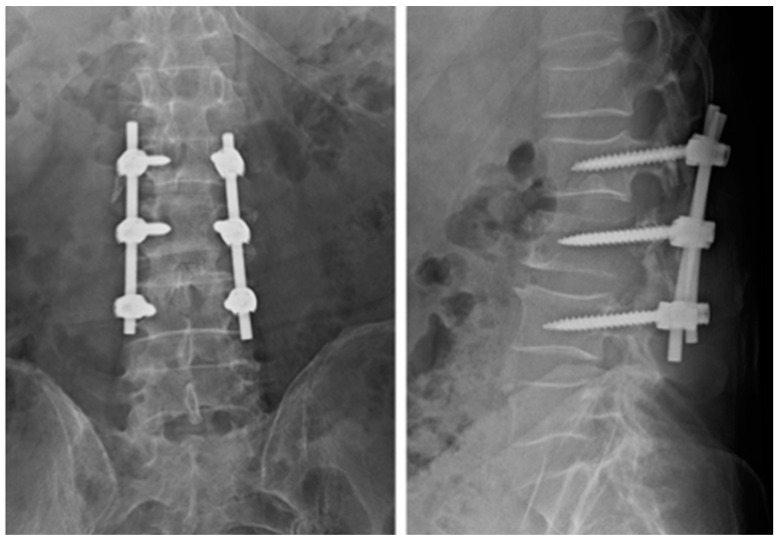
Case 1. Radiographs taken 3 months after surgery demonstrate no signs of implant loosening.
